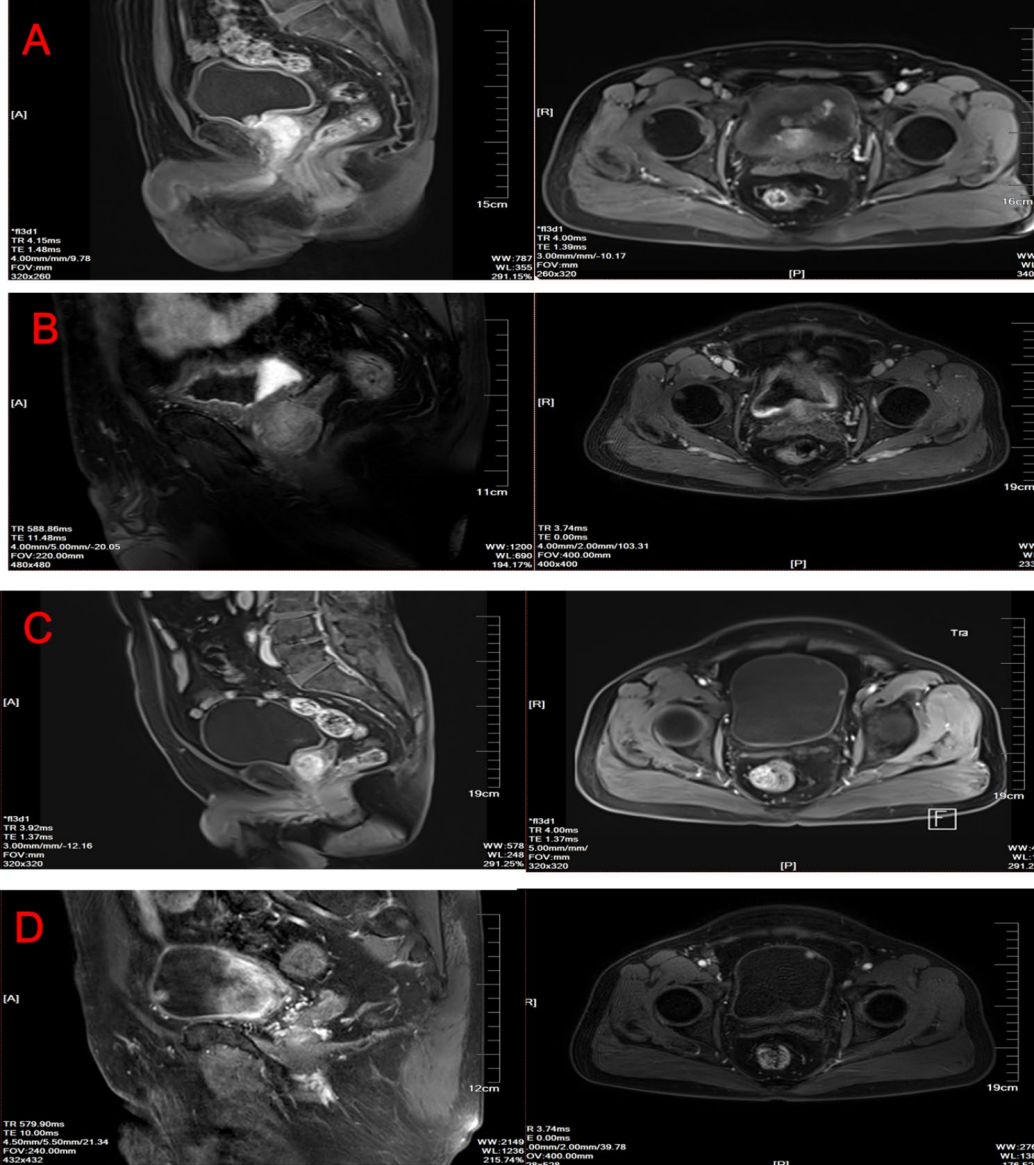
Magnetic resonance imaging of bladder cancer patient. A) MRI image revealed irregular and nodular enhancement, the largest which had a size of 2.9*1.2 cm in the bladder (sagittal and coronal) in November 2017. B) Pelvic MRI showing thickening of the posterior urinary bladder, and obvious intensification after enhancement (sagittal and coronal) in May, 2018. C) Pelvic MRI showing multiple nodular enhancement, the largest which had a size 0.6*0.8 cm in the bladder (sagittal and coronal) in November, 2018. D) Pelvic MRI showing signal abnormalities with the size of 0.9*0.7 cm in the bladder (sagittal and coronal) in June, 2019
